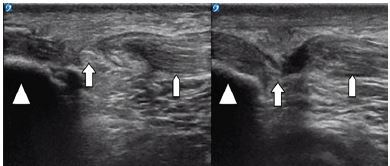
Ultrasound: the left and right correspond to the patient’s left and right patellar tendons. Orientation marker is directed toward the patient’s head and the probe is placed just at the inferior edge of the patella in a sagittal plane.
